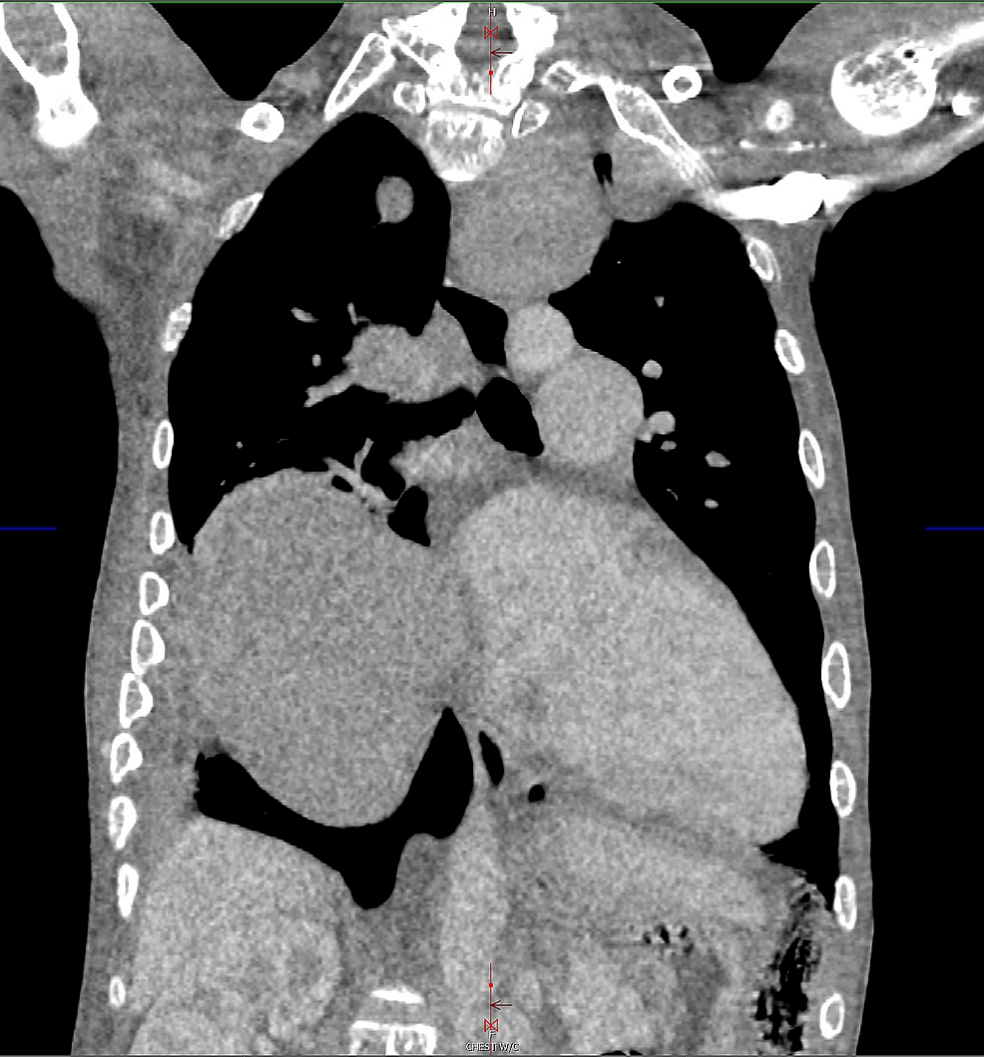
Computed Tomography of the Thorax: Coronal plane view demonstrates multiple soft tissue masses in the left hemithorax, as well as bilateral in the posterior paraspinal region, with the largest lesion on the right measuring over 9 cm in size.
